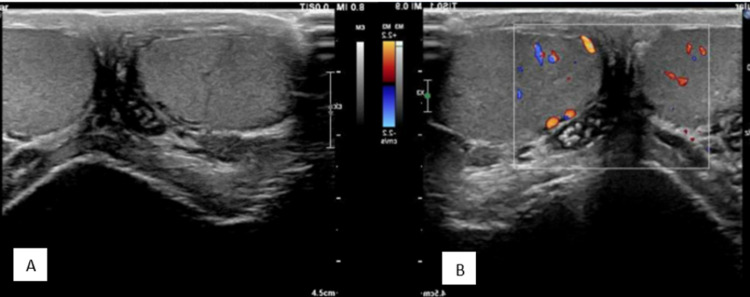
Doppler ultrasound of the testes showing (A) bilateral testes with normal echogenicity and (B) normal vascularization.
